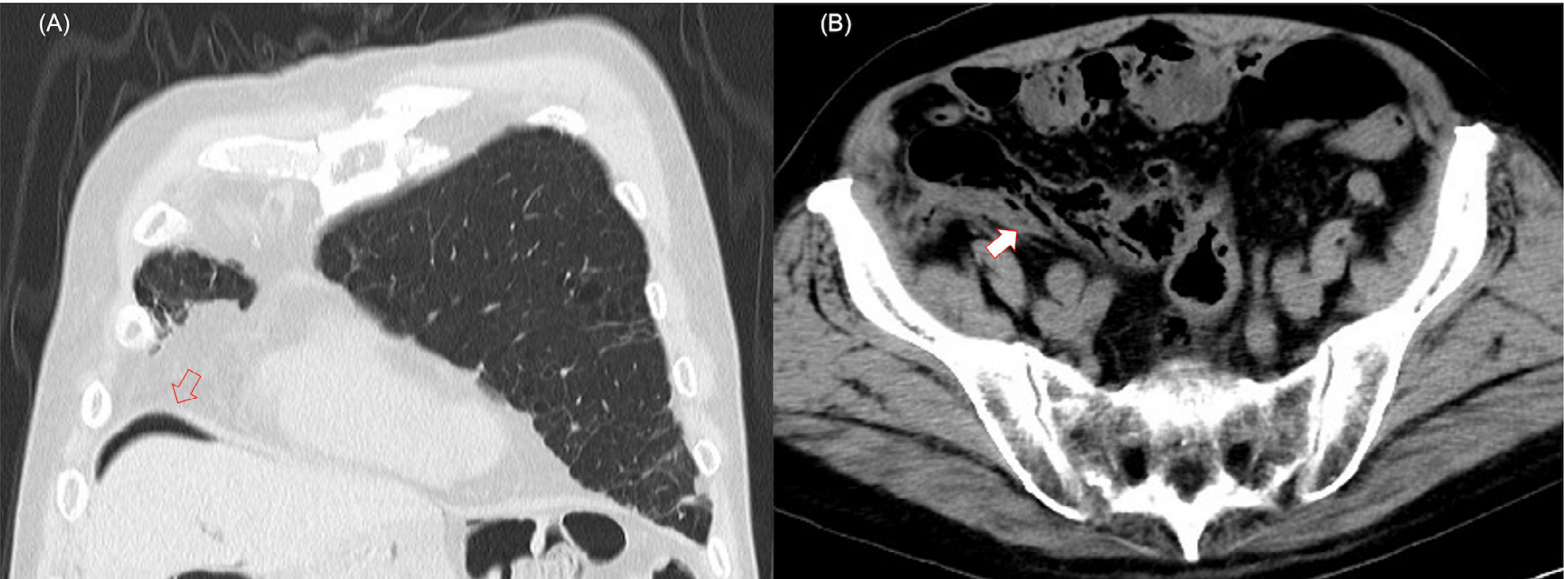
(A) Axial computed tomography lung window images at the development of free air in pneumatosis intestinalis (PI). The arrows show free air just below the diaphragm. (B) Transverse view of abdominal computed tomography: arrow shows PI in the intestinal wall
